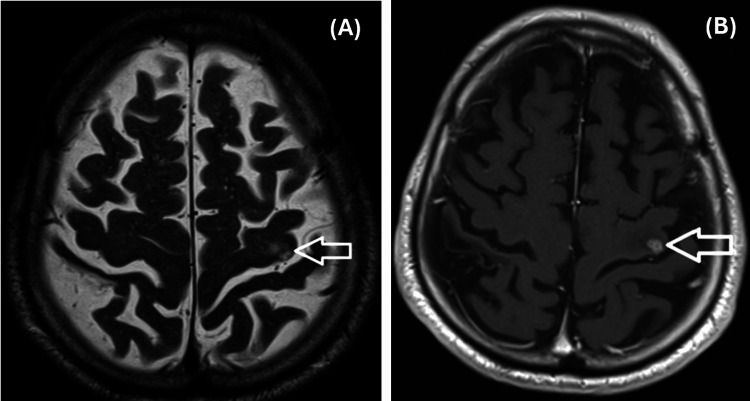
Baseline and post-SBRT MRI scans of the head (A) Baseline MRI scan depicting the solitary metastatic lesion in the left parietal lobe. (B) Post-SBRT scan depicting the solitary metastatic lesion in the left parietal lobe. SBRT, stereotactic body radiotherapy
